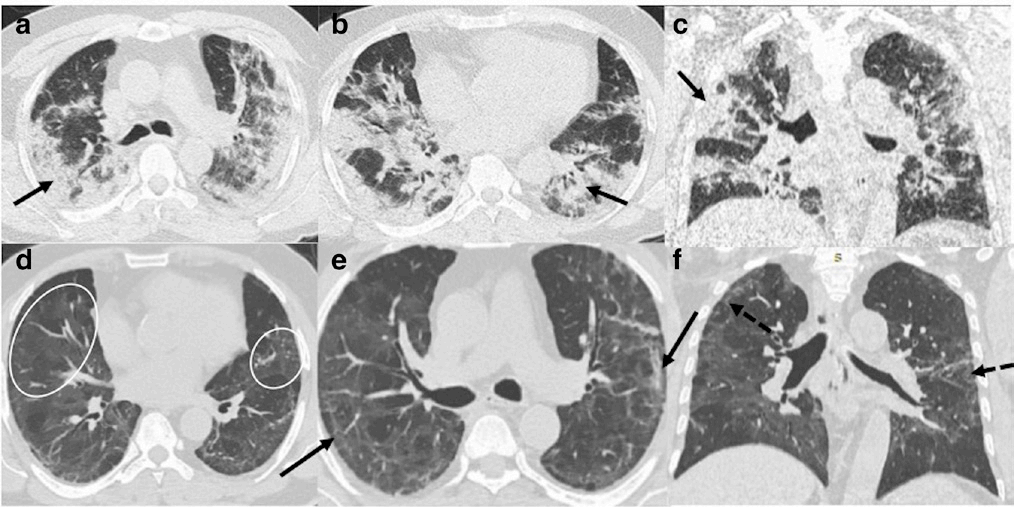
CT chest images of a 44-year-old male with persistent dyspnea showing
